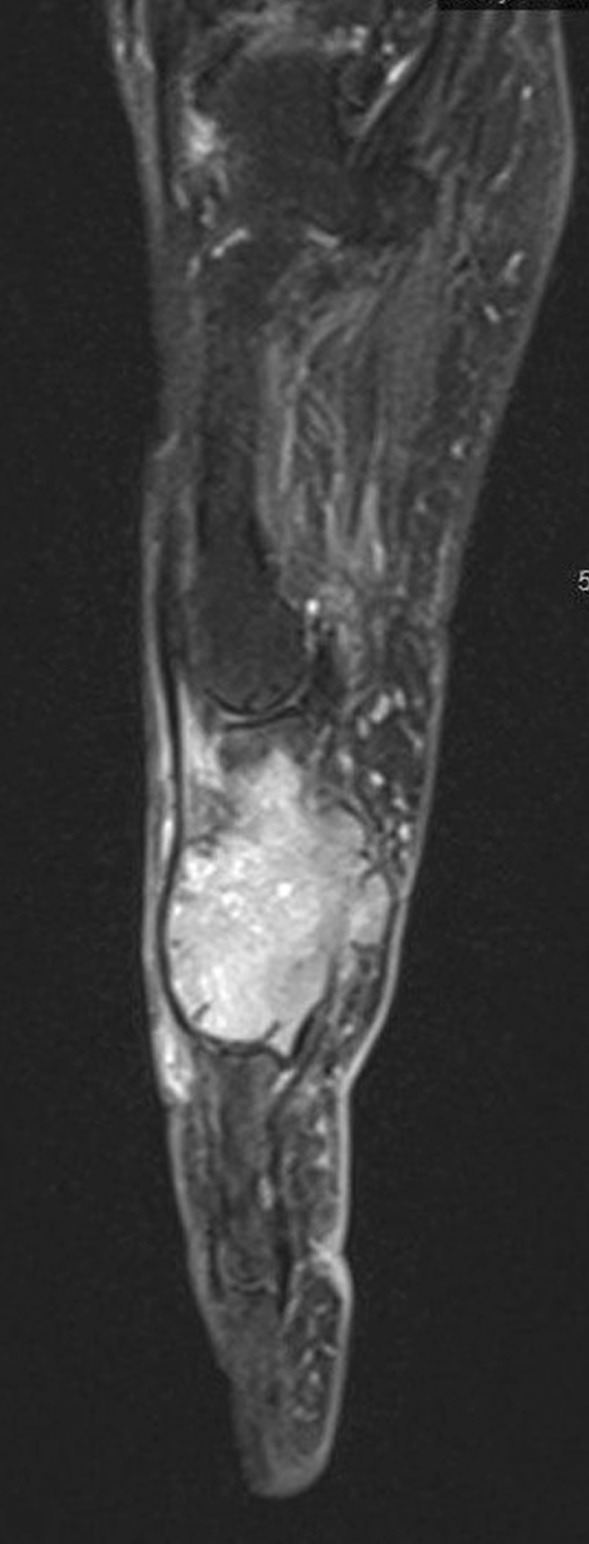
Fourth finger of the left hand, magnetic resonance imaging enhanced sagittal gadolinium T1-weighted section: section osteolytic lesion with the soft tissue component of the left fourth medial phalanx
